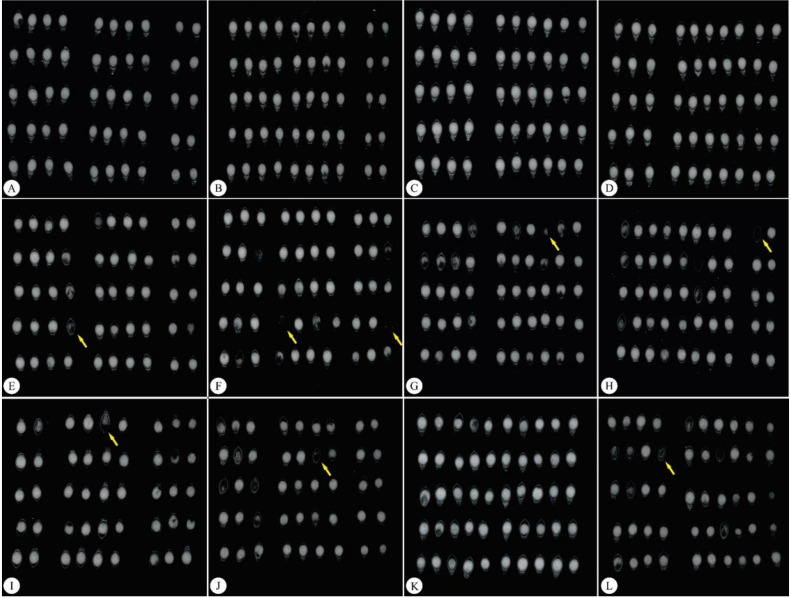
Differences in internal *Brachiaria brizantha* seed structures demonstrated by X-ray assessments. (A–D) Marandu cv. (E–H) Piatã cv. (I–L), Xaraés cv. Yellow arrows indicate damaged seeds.
